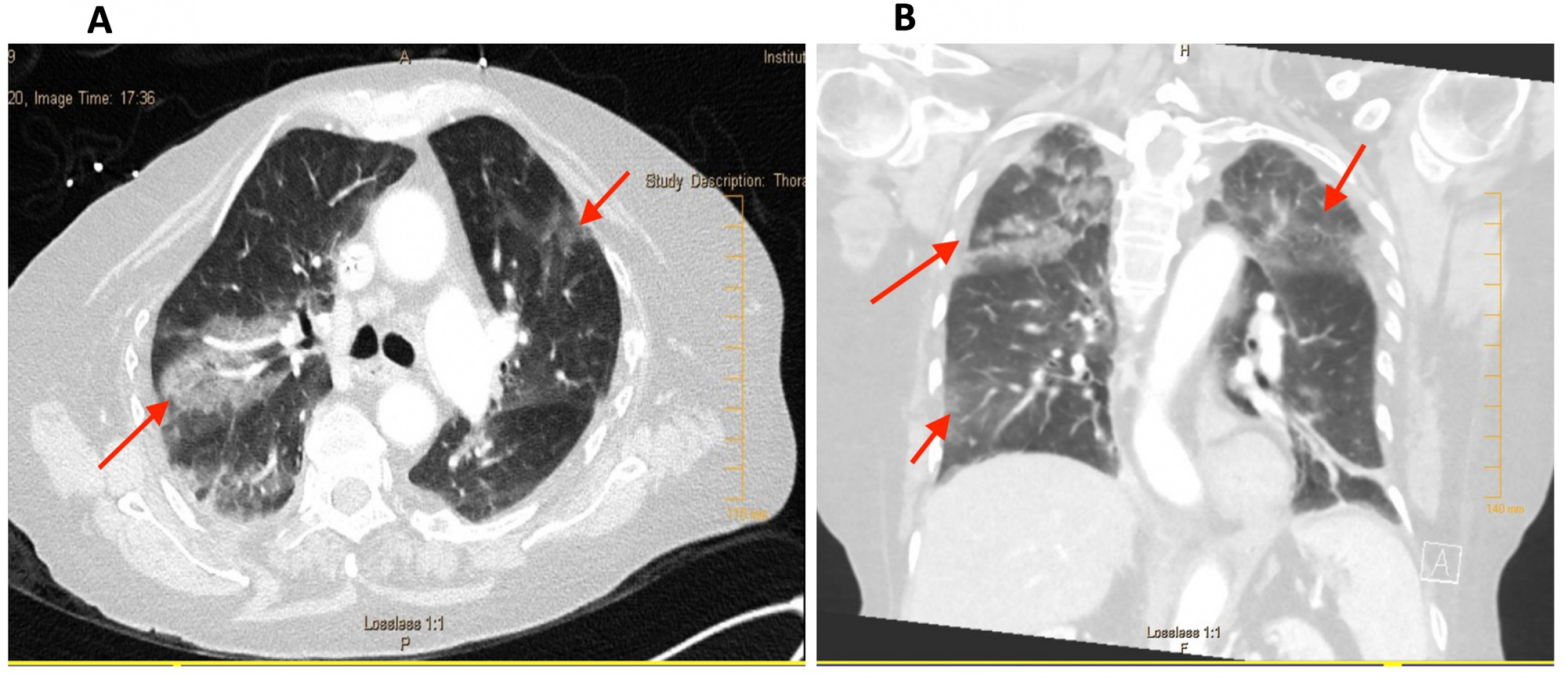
(A) Contrast-enhanced CT chest axial section (lung window) demonstrating patchy bilateral, right greater than left reticular and ground-glass airspace opacities, and (B) similar bilateral ground-glass opacities (coronal view)
